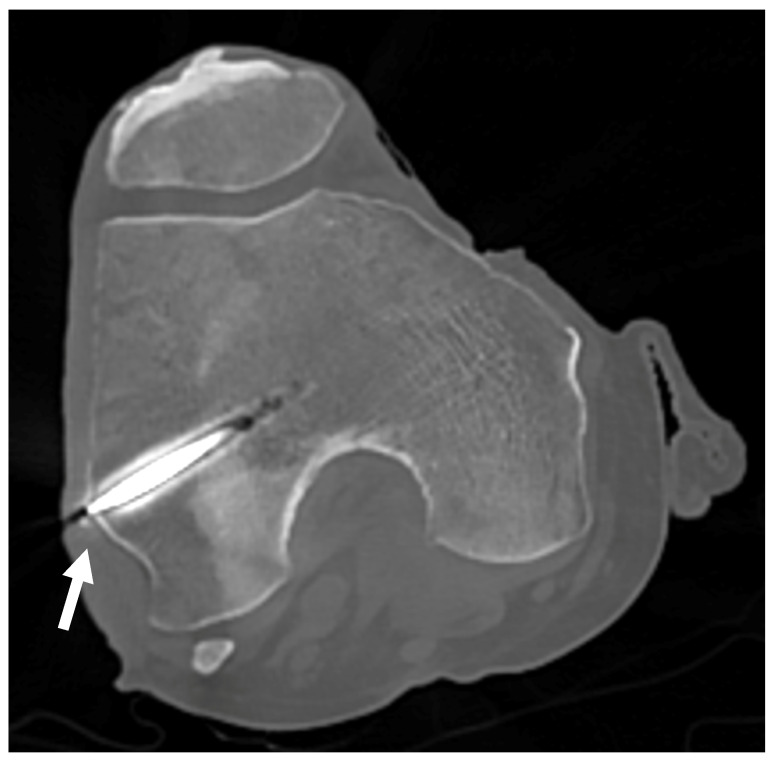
CT scan of knee 1. CT scan used to confirm proper positioning of the trocar for knees 1 to 4. The white arrow points to the LFE, confirming alignment with the targeted anatomical structure.
